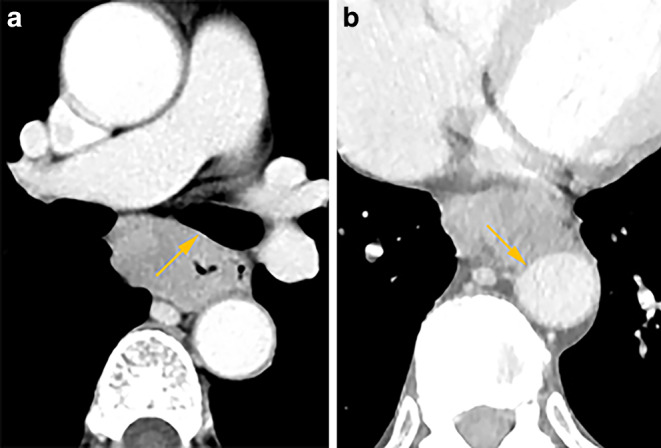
Typical CT images of borderline-resectable (a) and unresectable tumors (b). a shows the fatty plane between the primary tumor and left main bronchus to be blurred. b shows that the aorta is encased rigidly by a tumor with an interface arc between the tumor and aortic wall ≥ 90°
